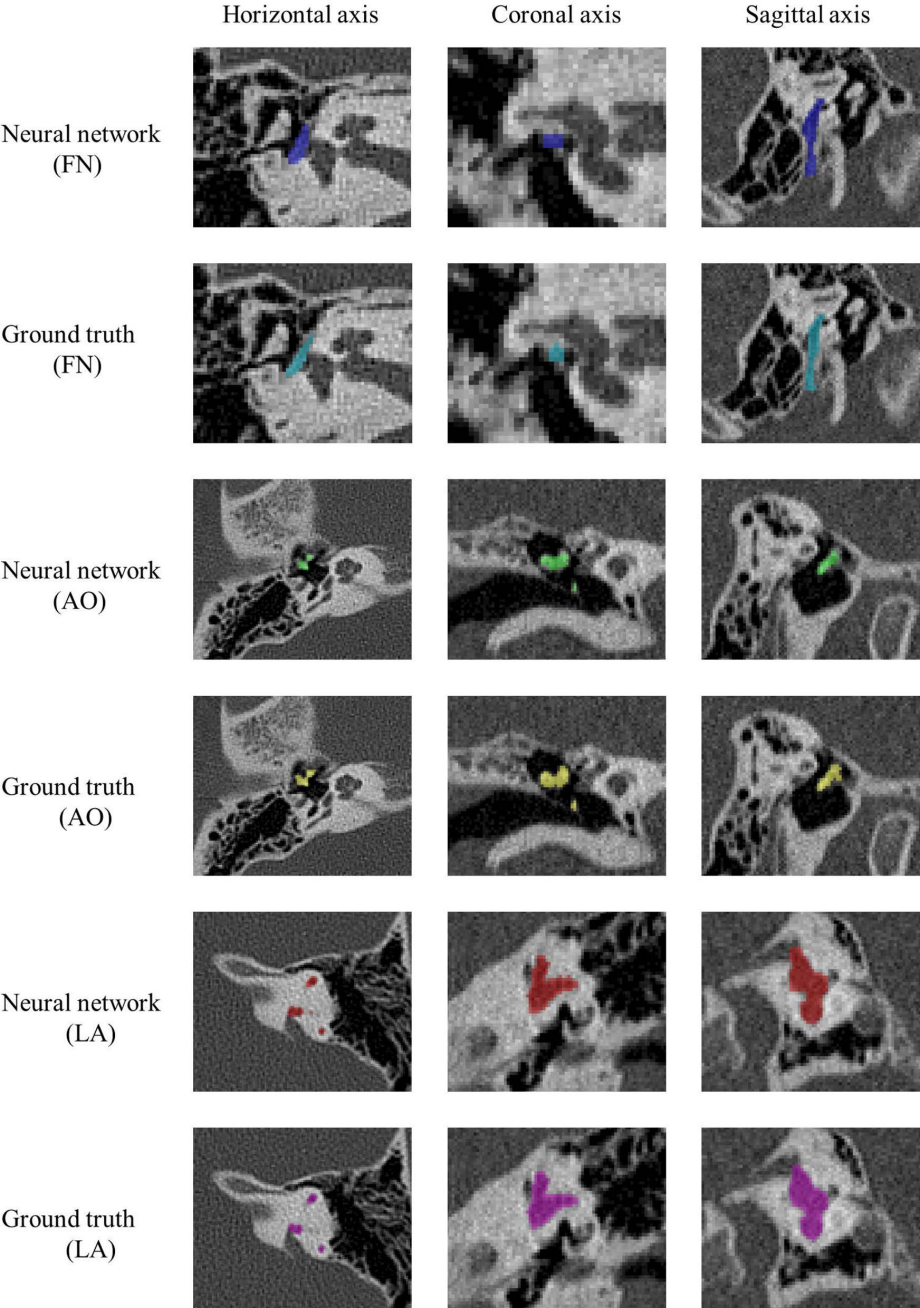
The masks of malformed structures created by two methods in abnormal temporal bone CT images. Blue, green, and red labels denote the FN (covering the vestibular window), AO (disruption), and LA (Mondini dysplasia), respectively. Turquoise, yellow, and magenta labels represent the manual segmentation of the FN (covering the vestibular window), AO (disruption), and LA (Mondini dysplasia), respectively. CT, computed tomography; FN, facial nerve; AO, auditory ossicle; LA, labyrinth
